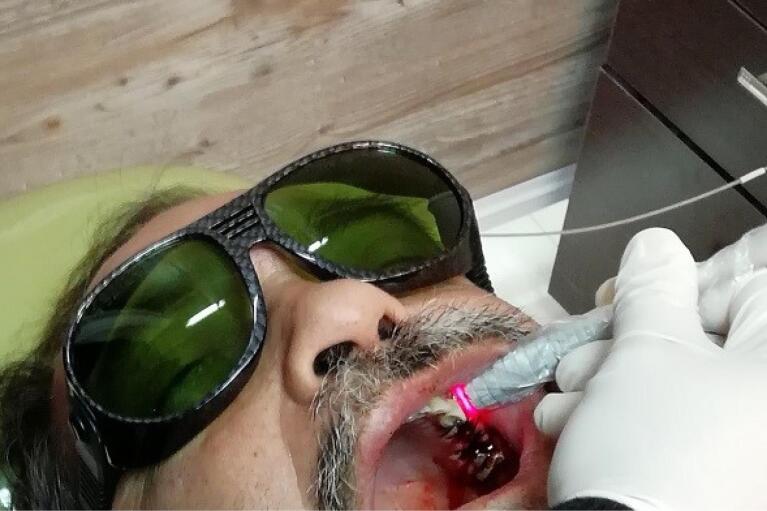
Bone quantification was performed at the implant site using periapical intraoral radiography with a parallel technique using a film holder to minimize photo distortion (Rinn XCP, Dentsply). Radiographic evaluation was performed twice, immediately after implant placement and after six months. The position of the film was also recorded to ensure the reproducibility of the graphics during postoperative x-ray examinations by the Coltene Speedex system (Coltene, Switzerland) so that the same positional graphics could be obtained six months later. Periapical radiographs were obtained using a computerized scanner with specifications as follows: 600 dp and 250 gray scales scanned by Image Tool software to measure distances in digital photographs. Corresponding distances were measured while using the software.
